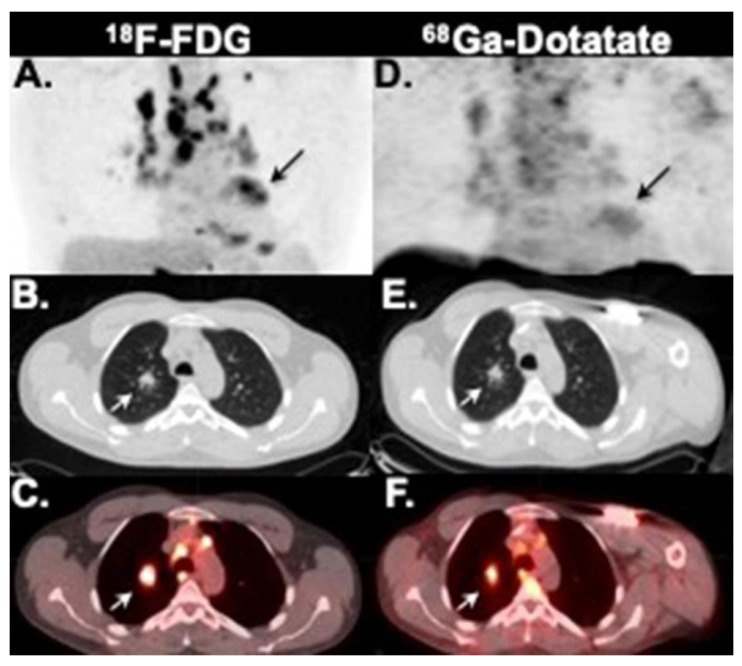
Patient with thoracic sarcoidosis. Both FDG and DOTATE PET images show extensive lymphadenopathy and cardiac inflammation (arrow on maximum intensity projections A,D) Axial CT (B,E) and fused PET-CT (C,F). Reprinted with permission from ref. [23] Copyright 2019 American Society of Nuclear Cardiology.
